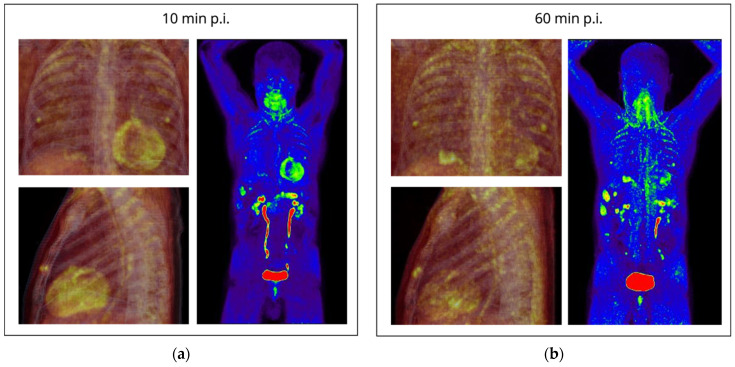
Example of a metastatic pancreatic ductal adenocarcinoma patient under distinct chemotherapy regimens (gemcitabine/nab-paclitaxel followed by modified FOLFIRINOX). (a) Early ^68^Ga-FAPI PET images 10 min p.i. (b) Late ^68^Ga-FAPI PET images 60 min p.i. Clockwise from upper-left: PET/CT fusion anterior view, PET/CT fusion lateral view, PET maximum-intensity-projection anterior view. Hybrid imaging shows the primary pancreatic carcinoma, multiple liver metastases, and peritoneal nodules (indicative of peritoneal carcinomatosis), each with intense FAP-expression. Chemotherapy-related cardiotoxicity was suspected due to increased cardiac FAP-expression (emphasized in early images p.i.) and incomplete cardiac wash-out (observed in late images). PET/CT images were acquired at our institution.
